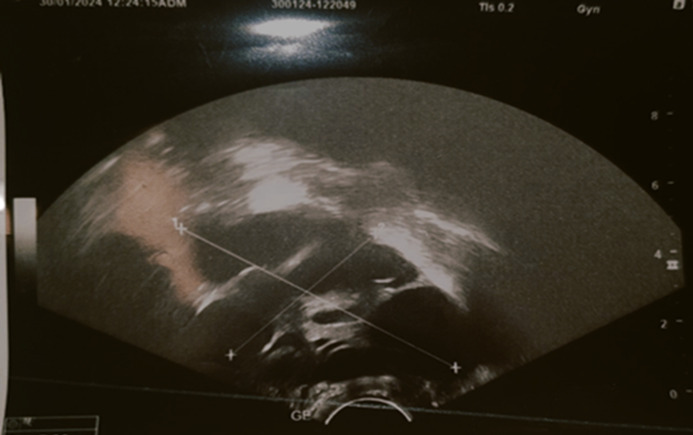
ultrasound appearance of enlarged, macrofollicular stimulated ovaries
